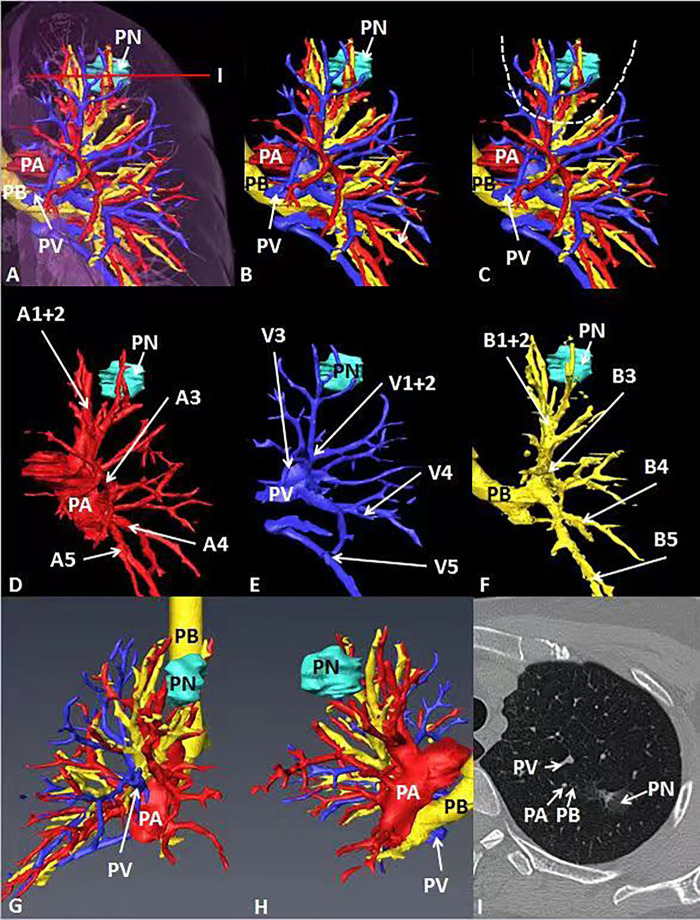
General CT, 3D-CT of the patient. (A–C) 3D-CT of the patient shows the location of pulmonary nodules in the left upper lung. (D–F) 3D-CT shows the position between target pulmonary nodules and surrounding pulmonary arteries, veins and bronchi. (G, H) Observe the position between target pulmonary nodules and surrounding pulmonary arteries, veins and bronchi from different perspectives. (I) The position between the target pulmonary nodule and the surrounding pulmonary artery, vein and bronchus on the General CT.
